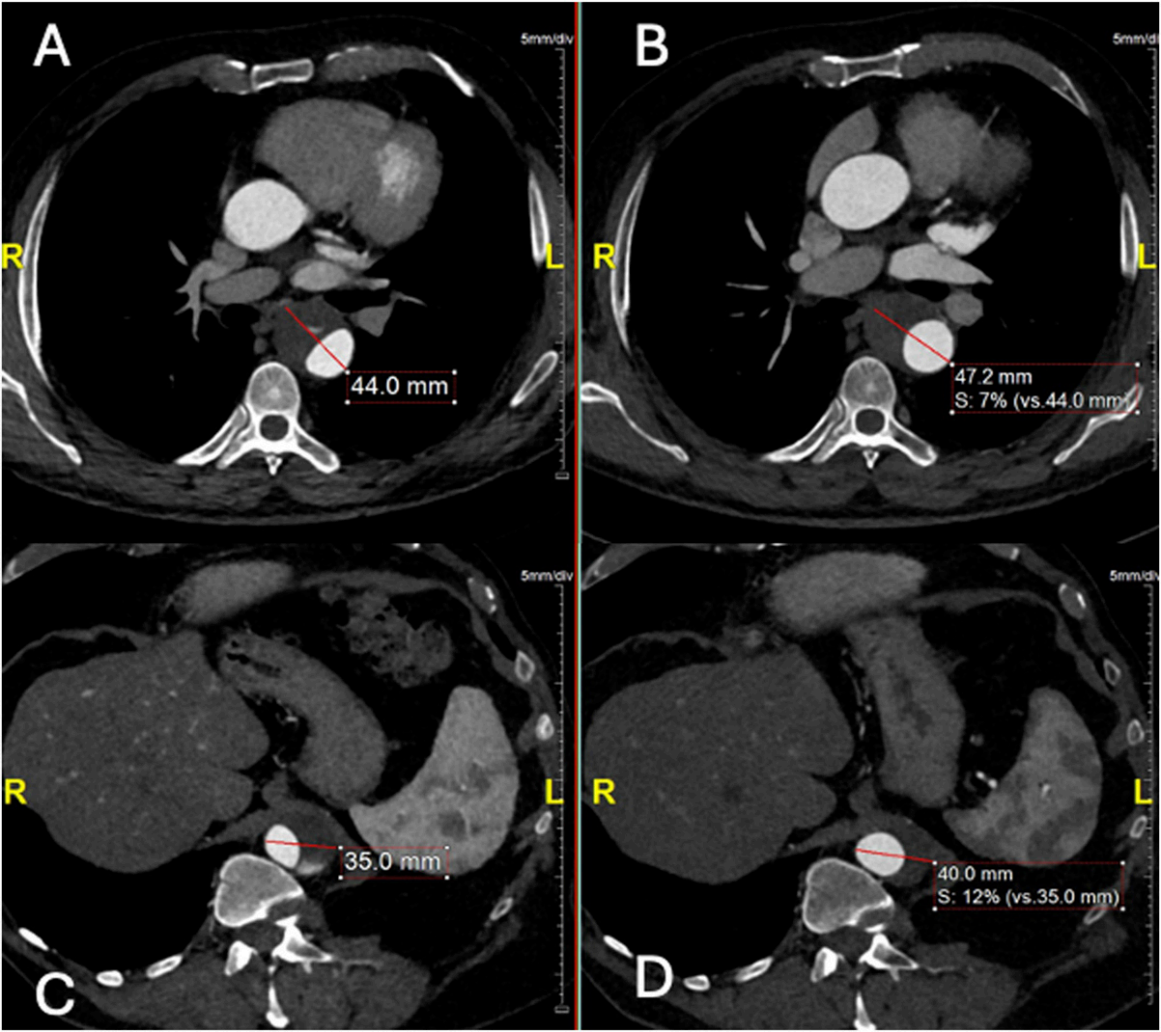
Aortic diameters comparison at 18 months follow-up. Axial CTA demonstrating (A) the diameter of thoracic aorta (44 mm) in April 2022, (B) the diameter of thoracic aorta (47.2 mm) in September 2023, (C) the diameter of abdominal aorta in April 2022 (35 mm), (D) the diameter of abdominal aorta (40 mm) in September 2023.
